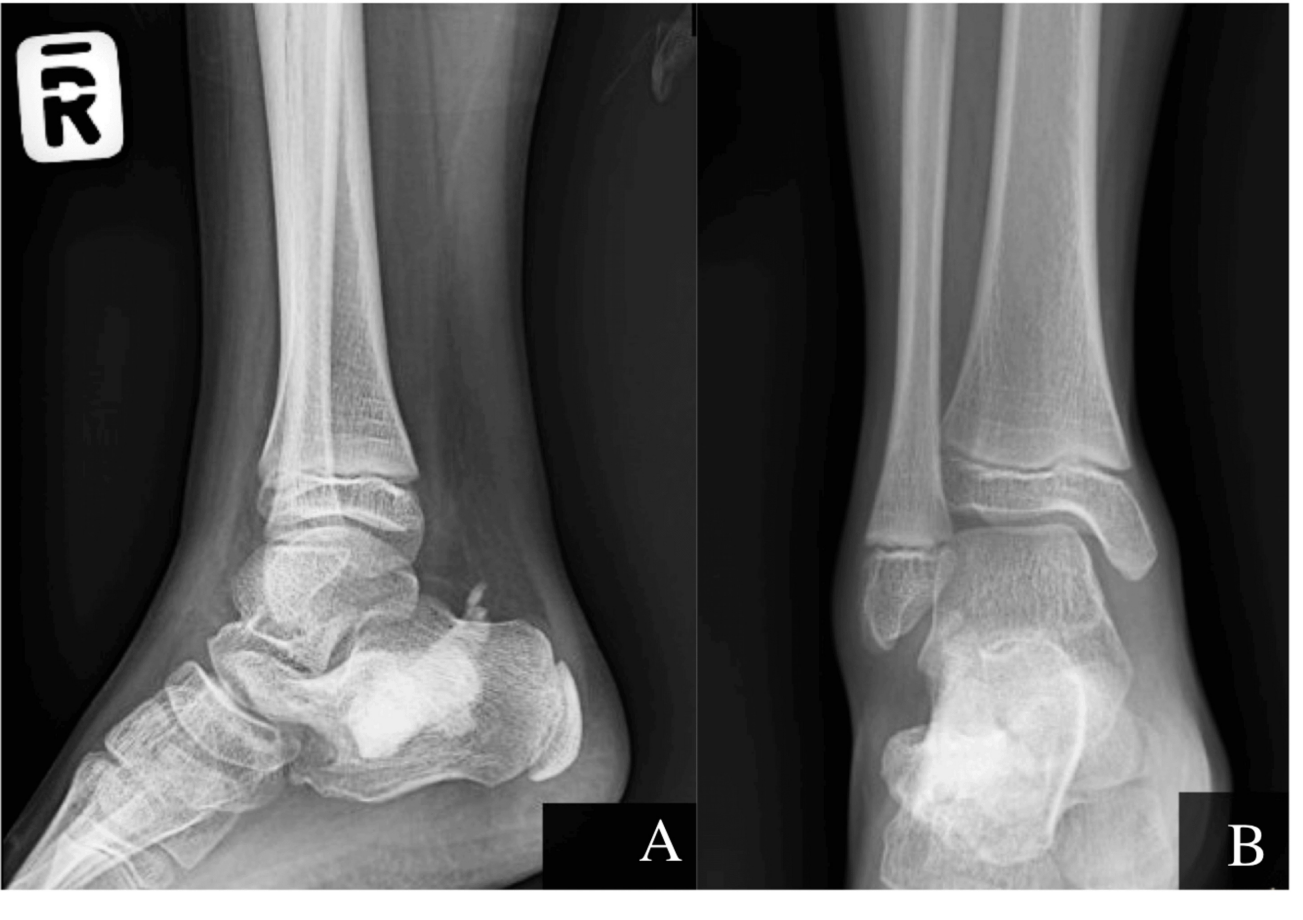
Postoperative radiographs in the lateral (A) and anteroposterior (B) views, showing the antibiotic bone cement spacer in place after 3 years
